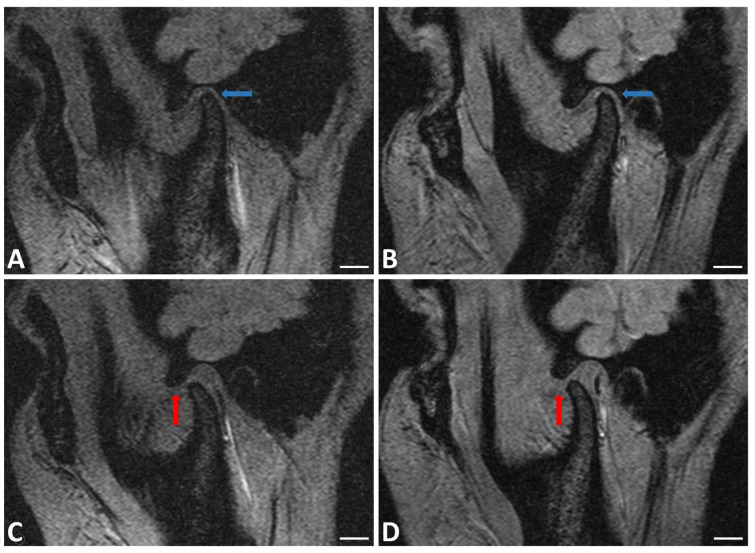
Right TMJ MRI in sagittal plane of patient with the jaw closed at T0 (A) and T1 (B) and open at T0 (C) and T1 (D). Increased disk and cartilage thickness (blue arrows) and increased excursion of mandibular condyle (red arrows) were observed. BAR = 1 cm
